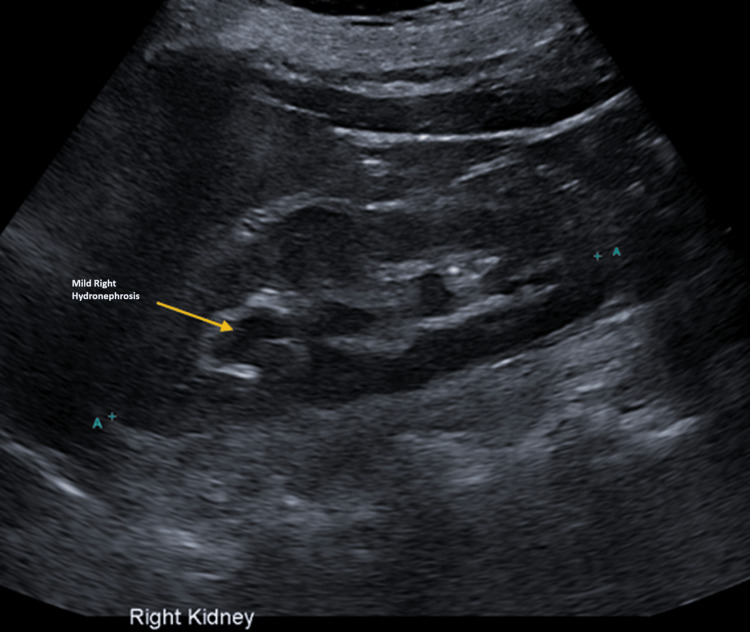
Ultrasound image of the right kidney demonstrating mild pelvicalyceal dilatation (hydronephrosis) (yellow arrow)
